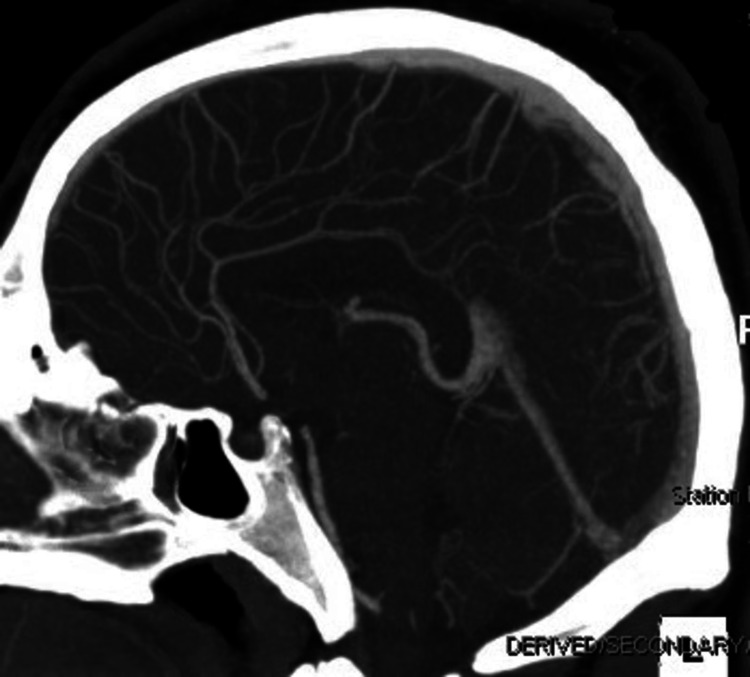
CT angiogram showing no acute large vessel occlusions
